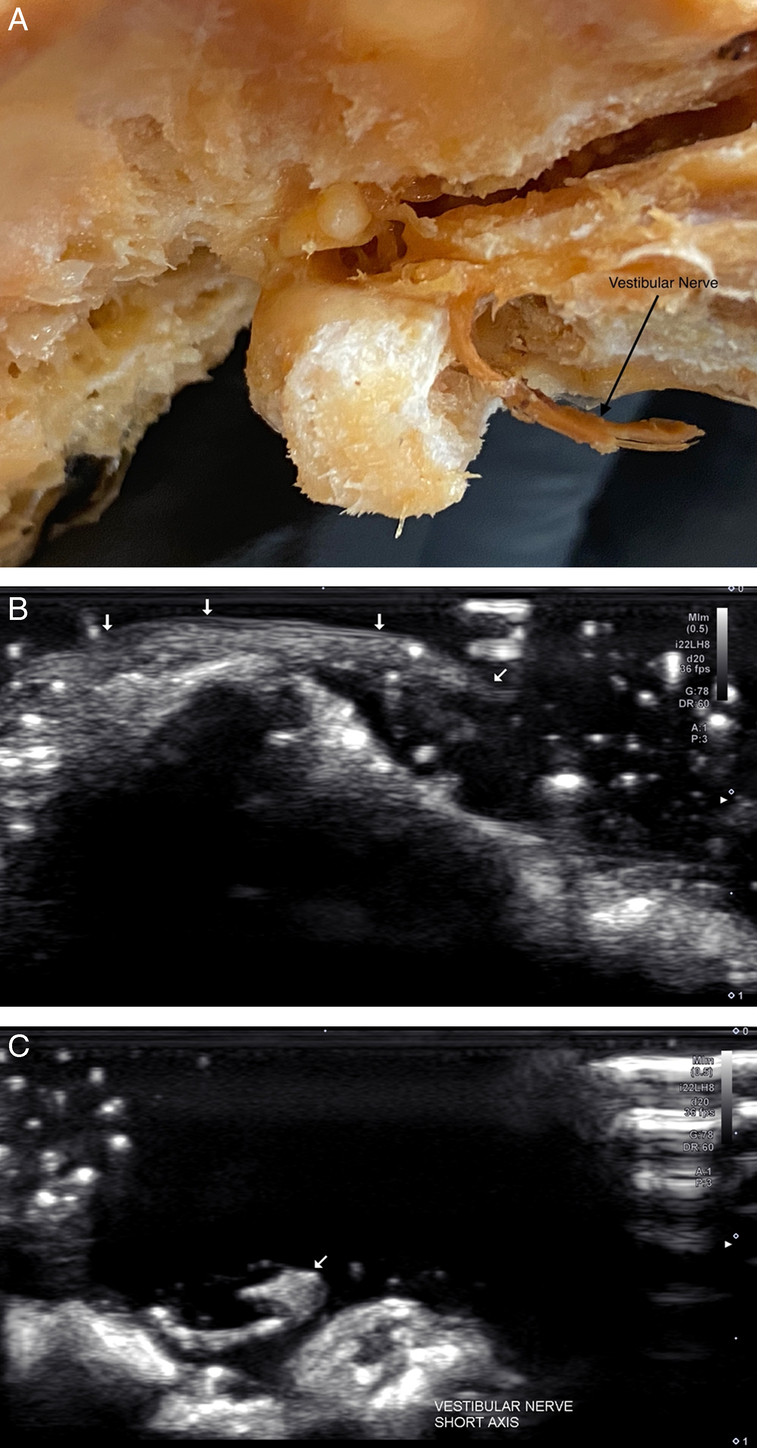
External appearance of the vestibular nerve, that, when identified sonographically, can be followed for localization of the utricle (A). A sonogram (22 MHz) of the vestibular nerve (white arrows) reveals the fascicular architecture in both long-axis (B) and short-axis (white arrow; C).
